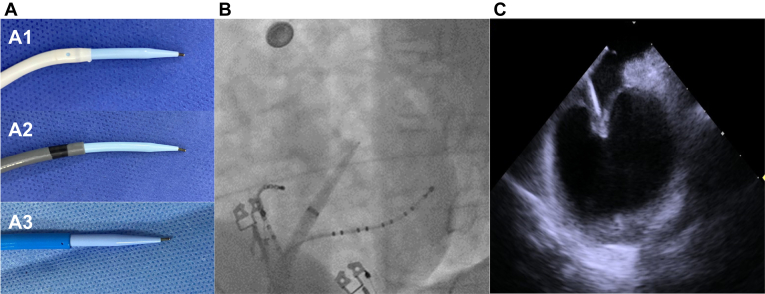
Performing the transseptal puncture. A: Sheaths with the J-tip of the wire positioned at the front passing through the atrial septum: nondeflectable sheath (A1), deflectable sheath (A2), cryoballoon sheath (A3). B: Fluoroscopy view of the J-tip passing through the atrial septum. C: Intracardiac echocardiography showing the J-tip passing through the atrial septum.
